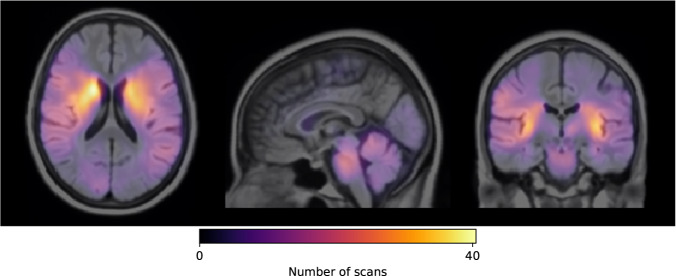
ISLES’22 probabilistic lesion map overlapped to an MNI FLAIR template.
